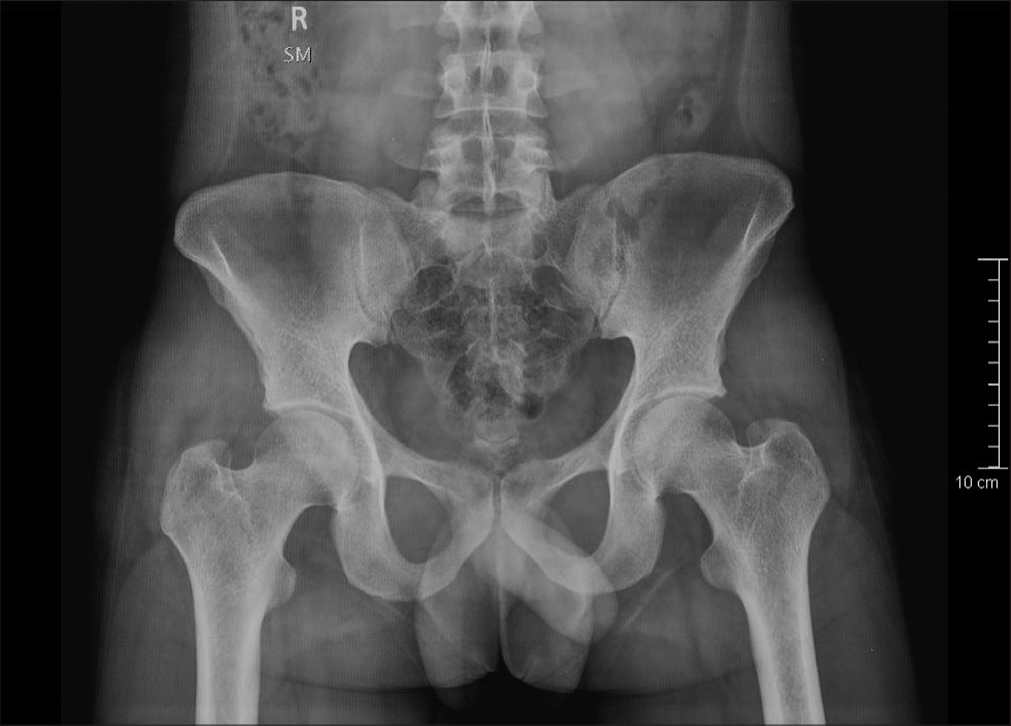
X-ray pelvis with both hip joints anteroposterior view showing no abnormality
